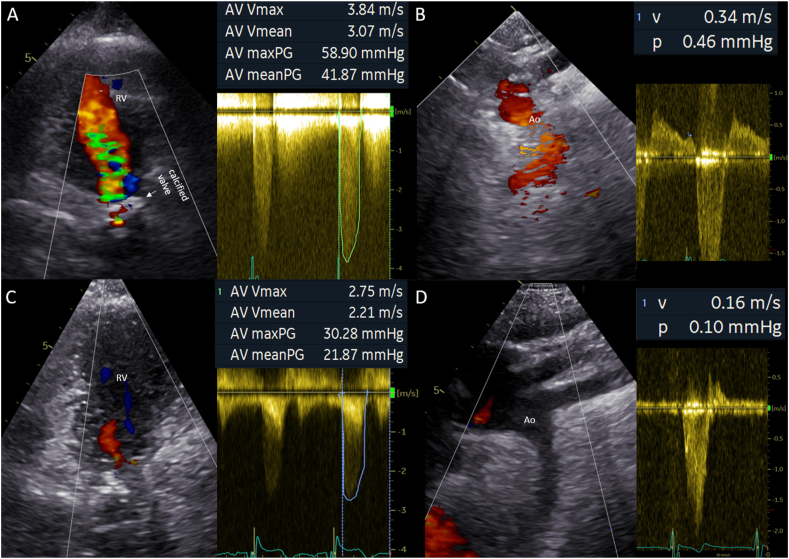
Transthoracic echocardiography imaging. Preprocedural: The modified apical two chamber view (A) diastolic frame showing the aortic homograft with severe regurgitation and severe stenosis (peak gradient 59 mmHg, mean gradient 42 mmHg). The suprasternal view (B) end-diastolic frame of the aortic arch with significant diastolic flow reversal in the descending aorta (0.34 m/s). Post-procedural, 3 months post re-intervention: The modified apical two chamber view (C) diastolic frame of the 20 mm Edwards Sapien 3 Ultra (Edwards Lifesciences, United Stated of America) bioprostheses in homograft with trace paravalvular regurgitation < grade 1 and normal gradient (peak pressure gradient 30 mmHg, mean pressure gradient 22 mmHg). The suprasternal view (D) end-diastolic frame of aortic arch with mild diastolic flow reversal (0.16 m/s). Ao: aorta, RV: right ventricle.
